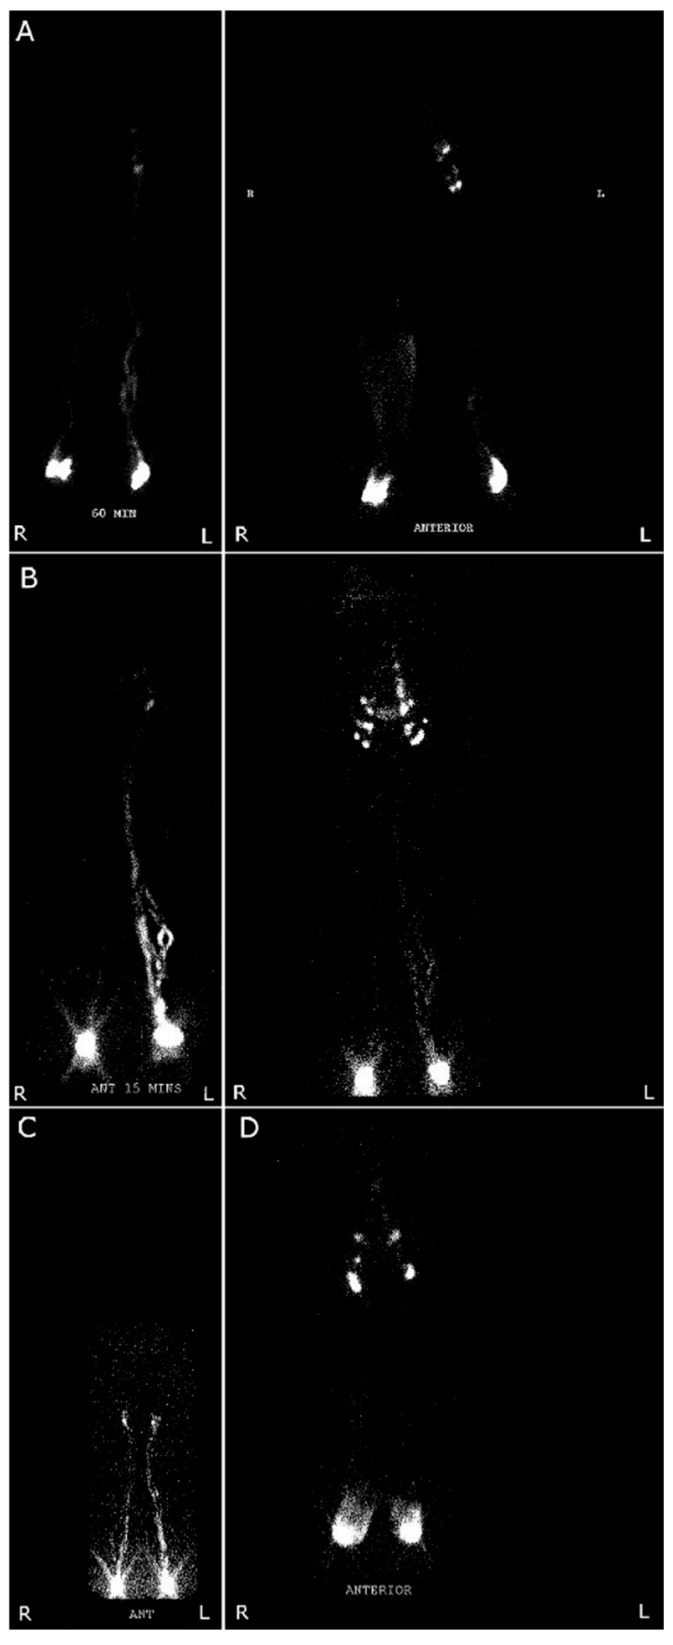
Anterior, lower body lymphoscintigraphy appearance of diseases that may be mistaken for lymphedema. (A) Lymphedema: delayed images (at 60 min and 5 h) with dermal backflow within the first hour and reduced visualization of lymphatics in the right leg. (B) Venous insufficiency with compensatory lymphatic activity: Images at 15 min and 4.5 h with immediate lymphatic drainage in both legs and more intense signal on the left in the setting of left-sided venous disease, suggesting. (C) Lipedema: Immediate image with slow transit time, without dermal backflow or structural abnormalities. (D) Obesity-induced lymphedema: Delayed image (4.5 h) with dermal backflow locally (greater on the right) and no evidence of structural or anatomic lymphatic abnormality. Abbreviations: L, Left; R, Right.
